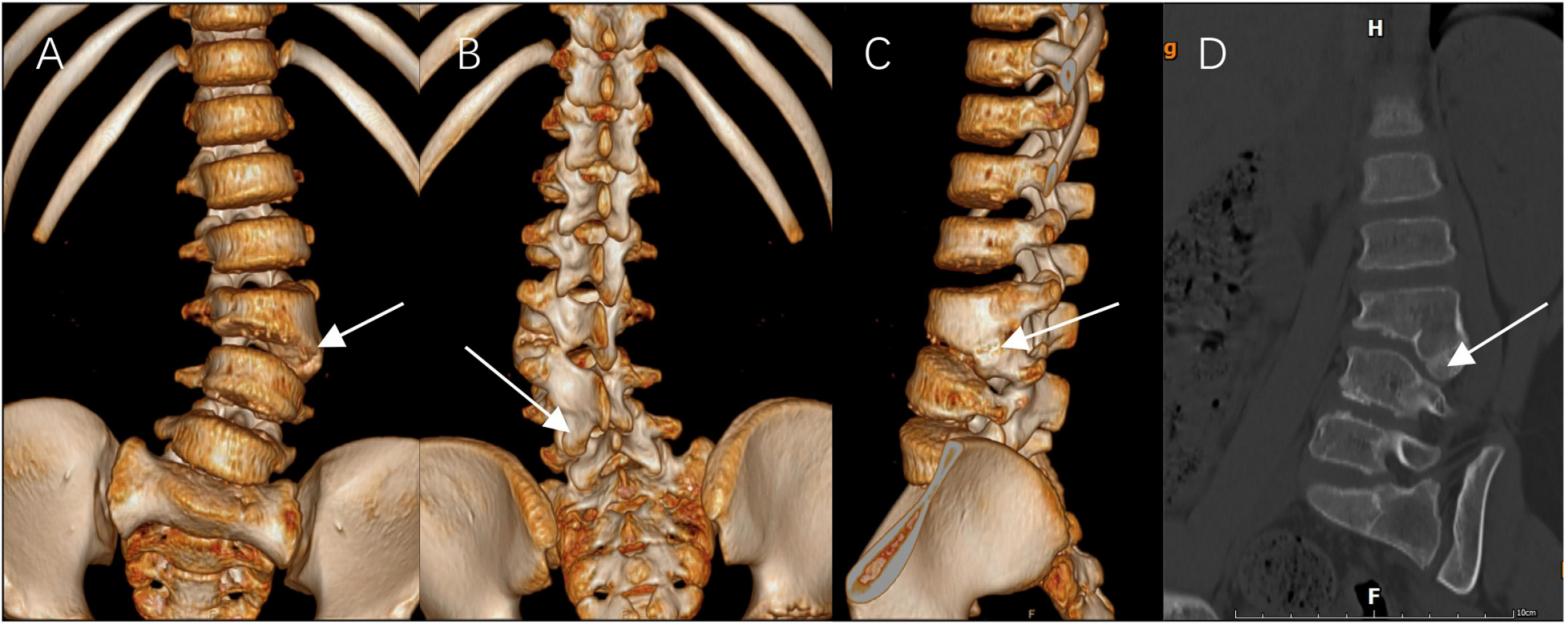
Preoperative 3D CT reconstructions of the L3/L4 HV (white arrow). (A) Anterior view. (B) Posterior view. (C) Left lateral view. (D) Sagittal cut view.
